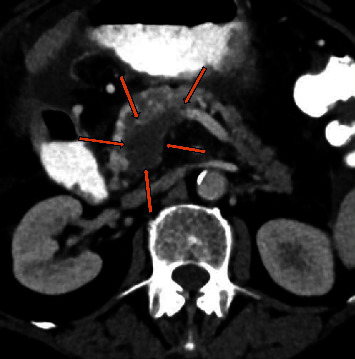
Computerized tomography (CT scan) portal vein phase, transverse section: a nonenhanced thrombus in the portal vein is depicted, indicating a chronic phase (orange arrows).
